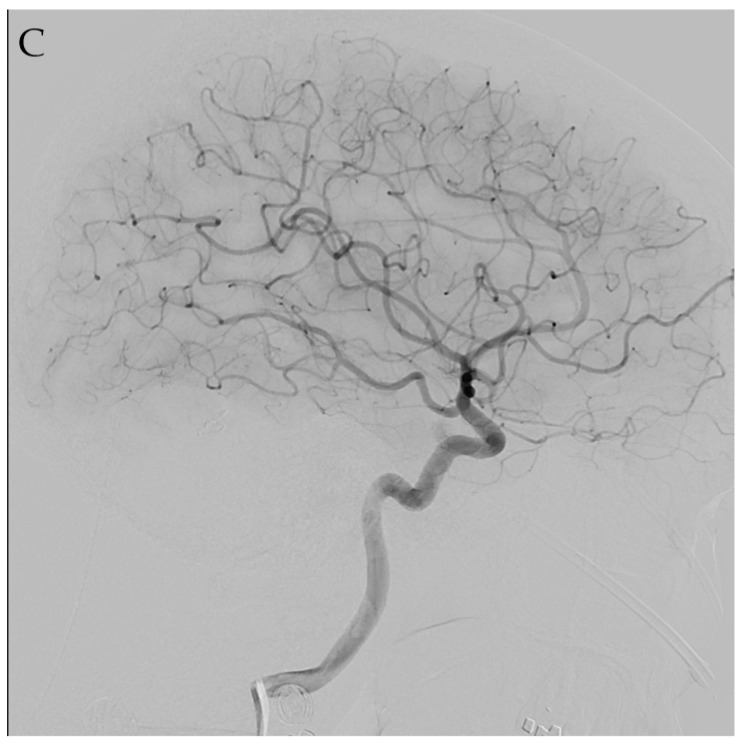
A 47-year-old female who presented with headaches and dizziness found to have an unruptured left anterior frontal lobe SM grade I AVM on MRI (A) with arterial supply from an orbitofrontal branch of the anterior cerebral artery (ACA) with venous drainage via a cortical vein into the anterior superior sagittal sinus (B). The patient was presented with microsurgical resection, embolization, and SRS as treatment options. Given the risk of residual AVM after embolization and a latent period between SRS and AVM obliteration, the patient opted for microsurgical resection. Follow-up angiography demonstrated no residual lesion (C).
